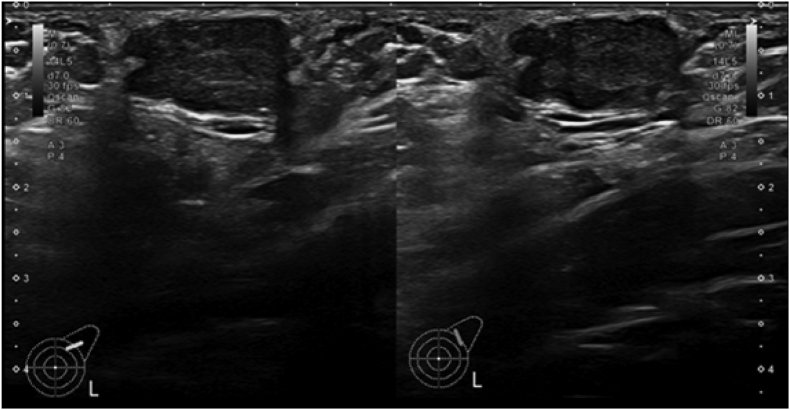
Ultrasound left axilla: 17.3 mm × 10.6 mm x 17.5 mm lobulated superficial hypoechoic well defined solid nodule with internal vascularity.
